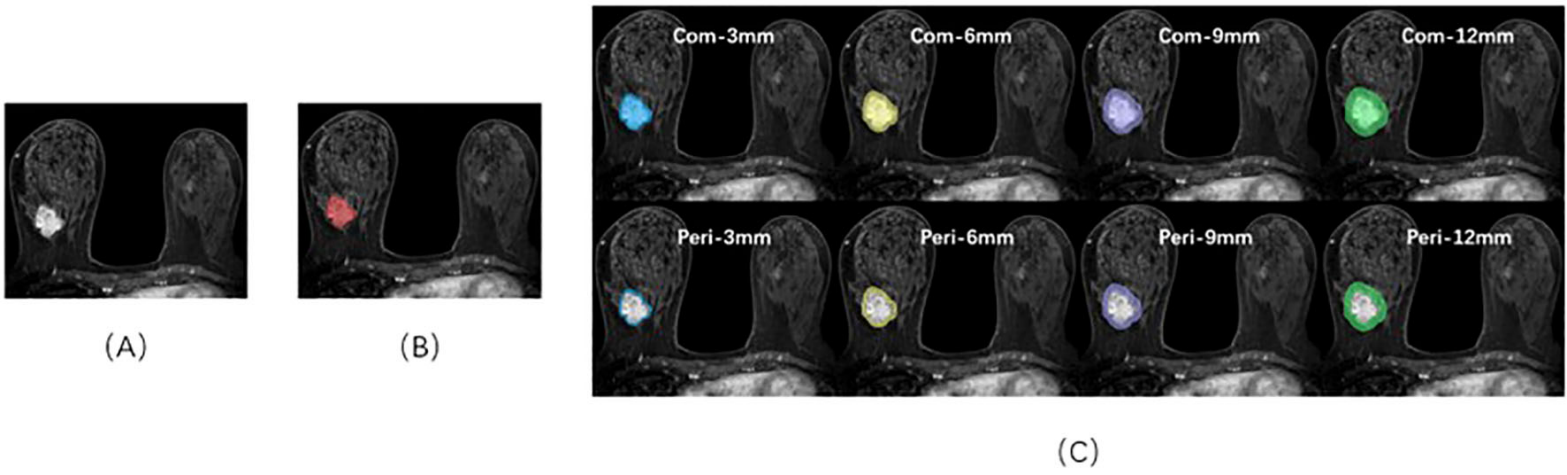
Sketch of the breast cancer ROI on DCE-MRI images using 3D-Slicer software. (A) A 46-year-old female patient with triple-negative breast cancer. (B) The red area represents the ROI of the tumor body. (C) ROIs of the tumor bodies and peritumoral and peritumoral regions in different ranges.
